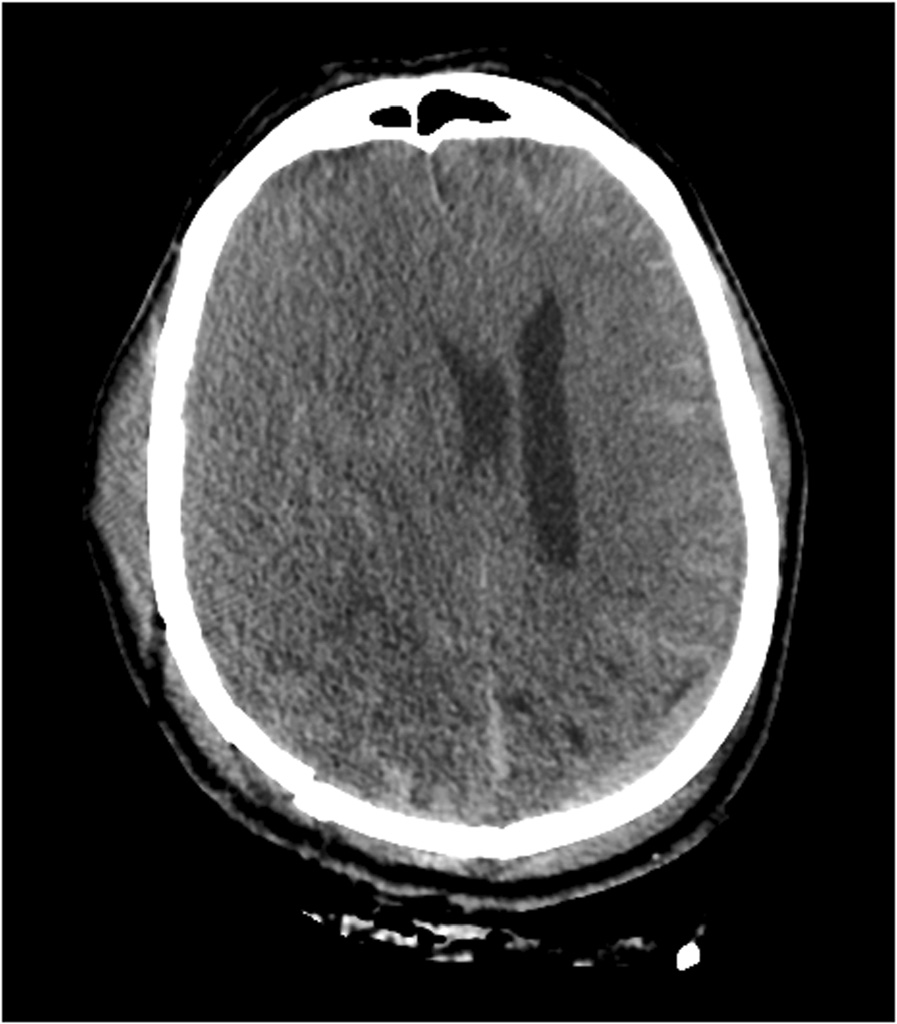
Cerebral computed tomography scan without contrast, axial section, parenchymal window. Homogeneous subdural isodense hematoma, right hemisphere, with deviation from the midline and contralateral subarachnoid hemorrhage
